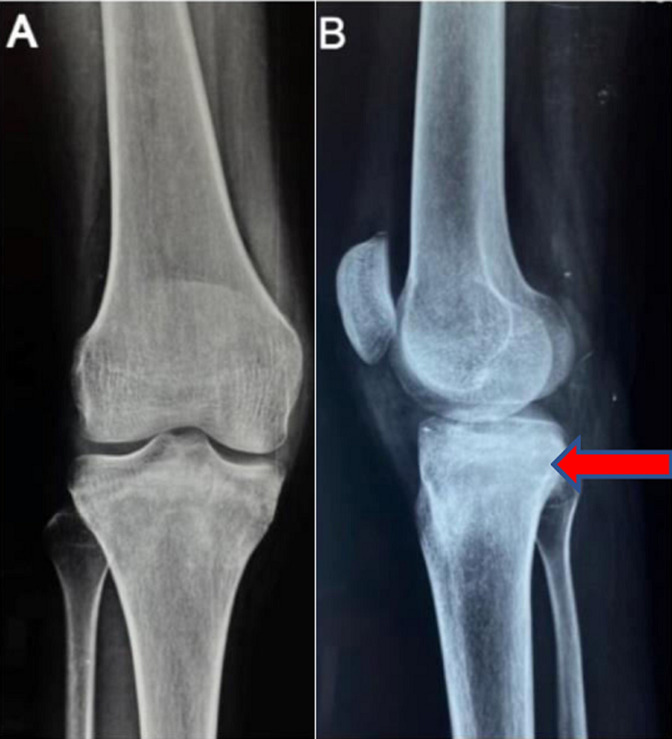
X-ray of right knee joint: A) the anterior-posterior view showing increased bone mineralisation and swelling of soft tissue over proximal tibial region; B) the lateral view showing well defined circular, lytic lesion over posterior aspect of proximal tibia
